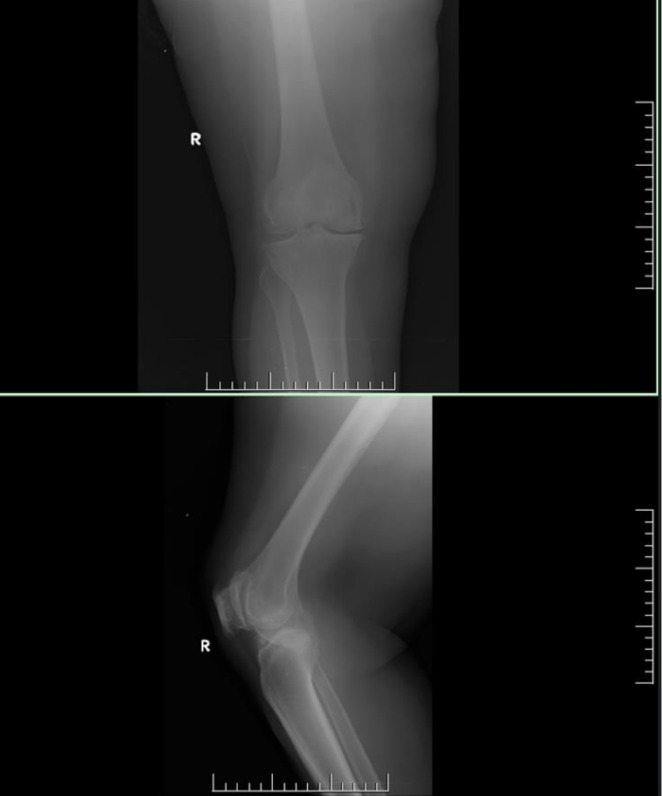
Radiographic image of the right knee, demonstrating reduced joint space.
